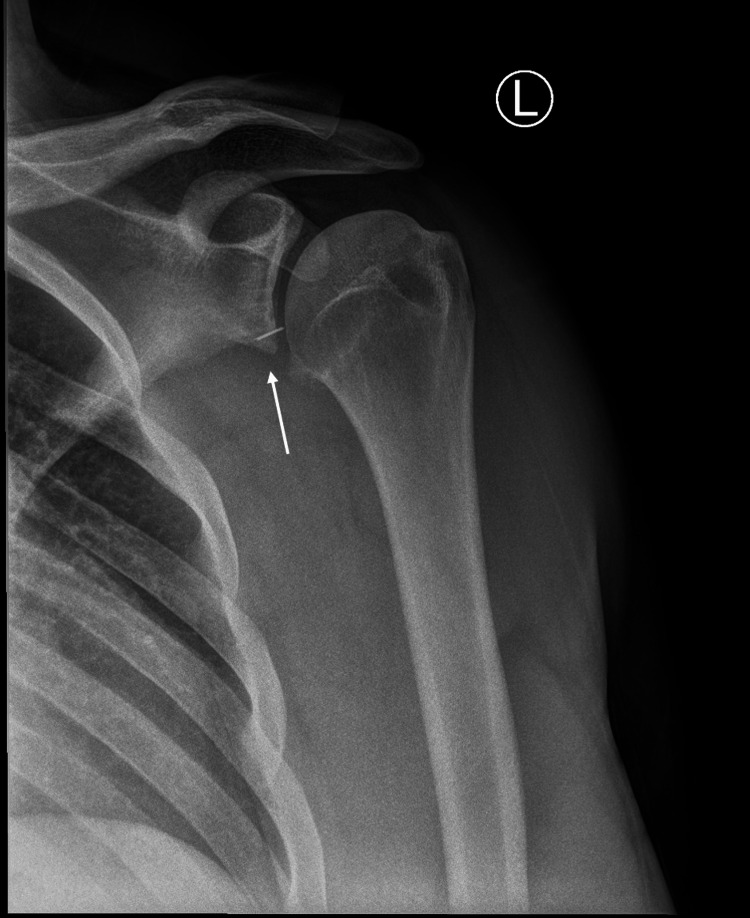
Grashey radiograph demonstrating retained metallic intraarticular body in the glenoid indicated by the arrow.
